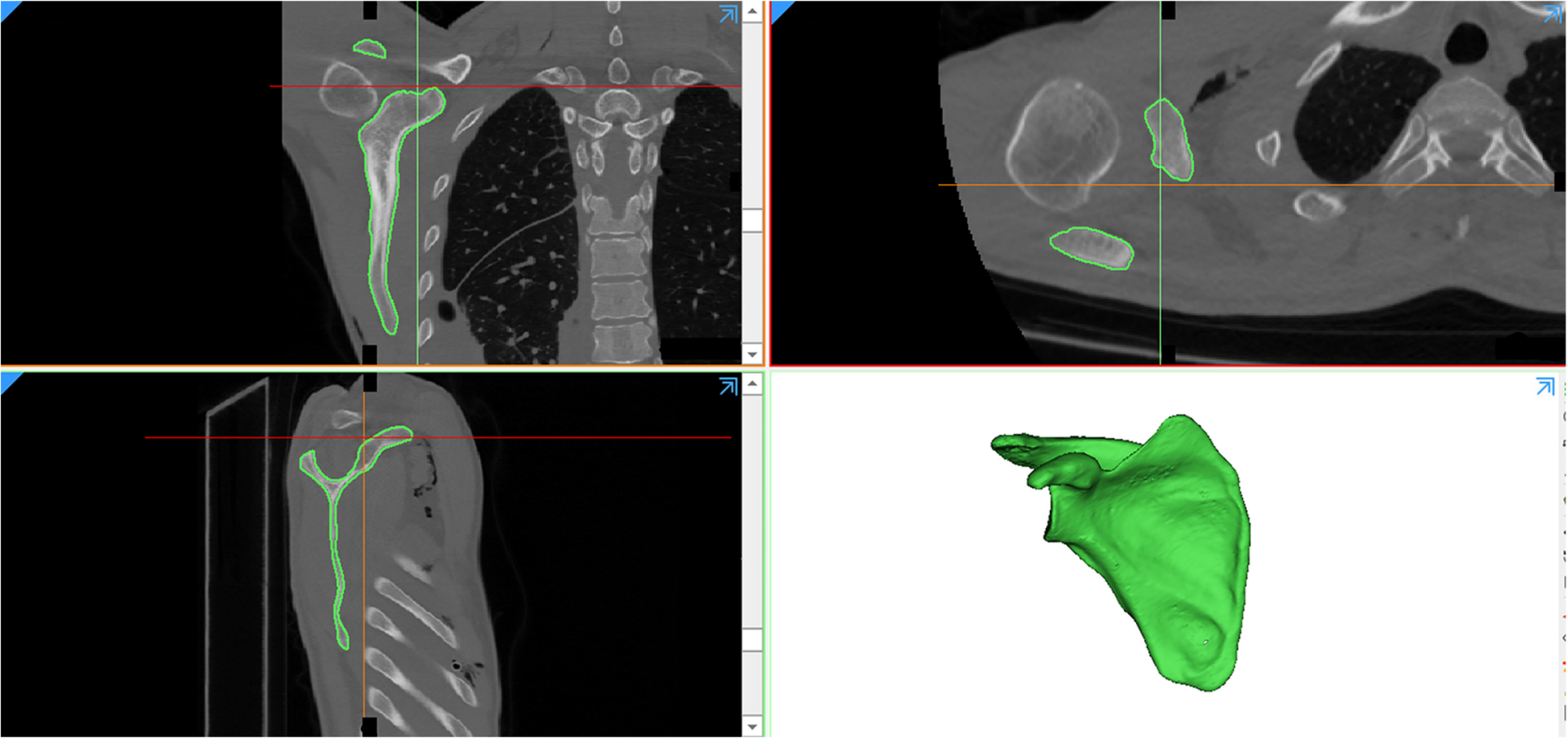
DICOM-formatted CT scan images were imported into Mimics software. Then, a right virtual scapula model was created by image segmentation, region growth and multiple slice editing
